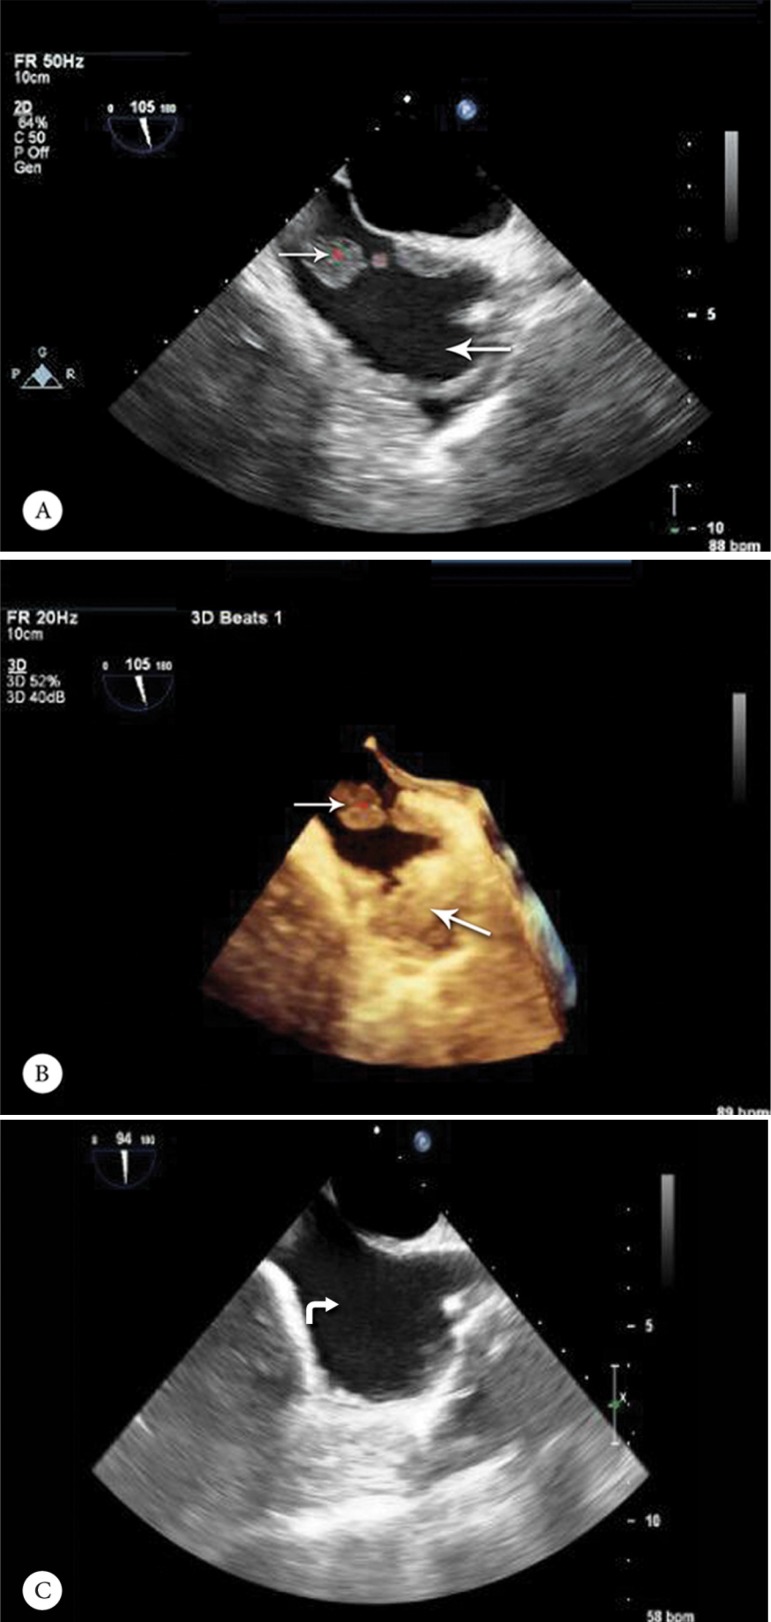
Transesophageal echocardiogram, bicaval view (Panels A and B), showing a large mass (narrow arrow) with 2 fixed (33×12 mm) and mobile (11 mm) components at the origin of the inferior vena cava (IVC) (thick arrow) entering into the right atrium. There was no residual thrombus in the IVC (curved arrow) during week 16 (Panel C).
